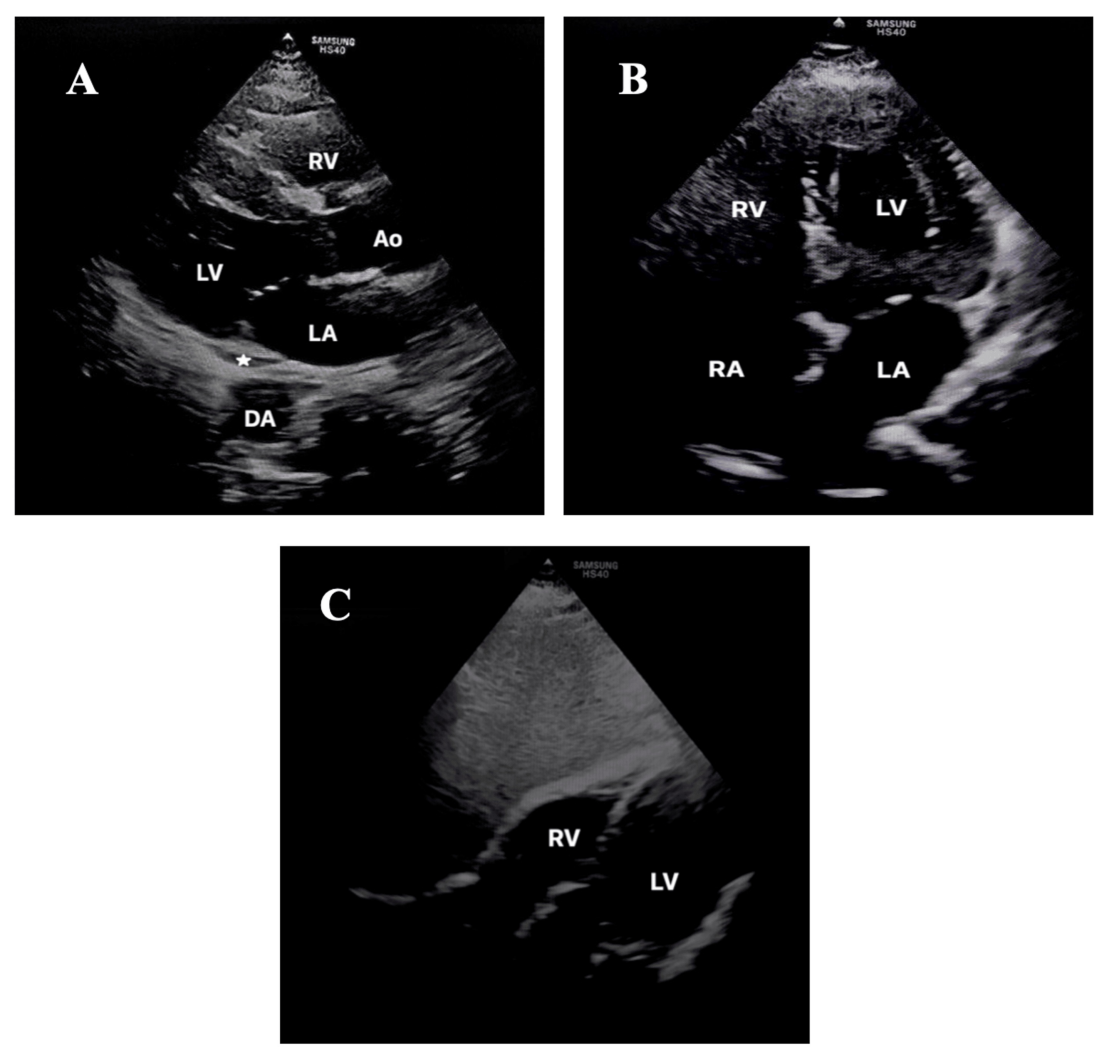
Repeated echocardiogram four months after levothyroxine treatment revealing a significant reduction of pericardial effusion, as depicted by the white star: (A) Parasternal long axis; (B) Apical four-chamber view; (C) Subcostal view Ao: aorta; DA: descending aorta; LA: left atrium; LV: left ventricle; RA: right atrium; RV: right ventricle
